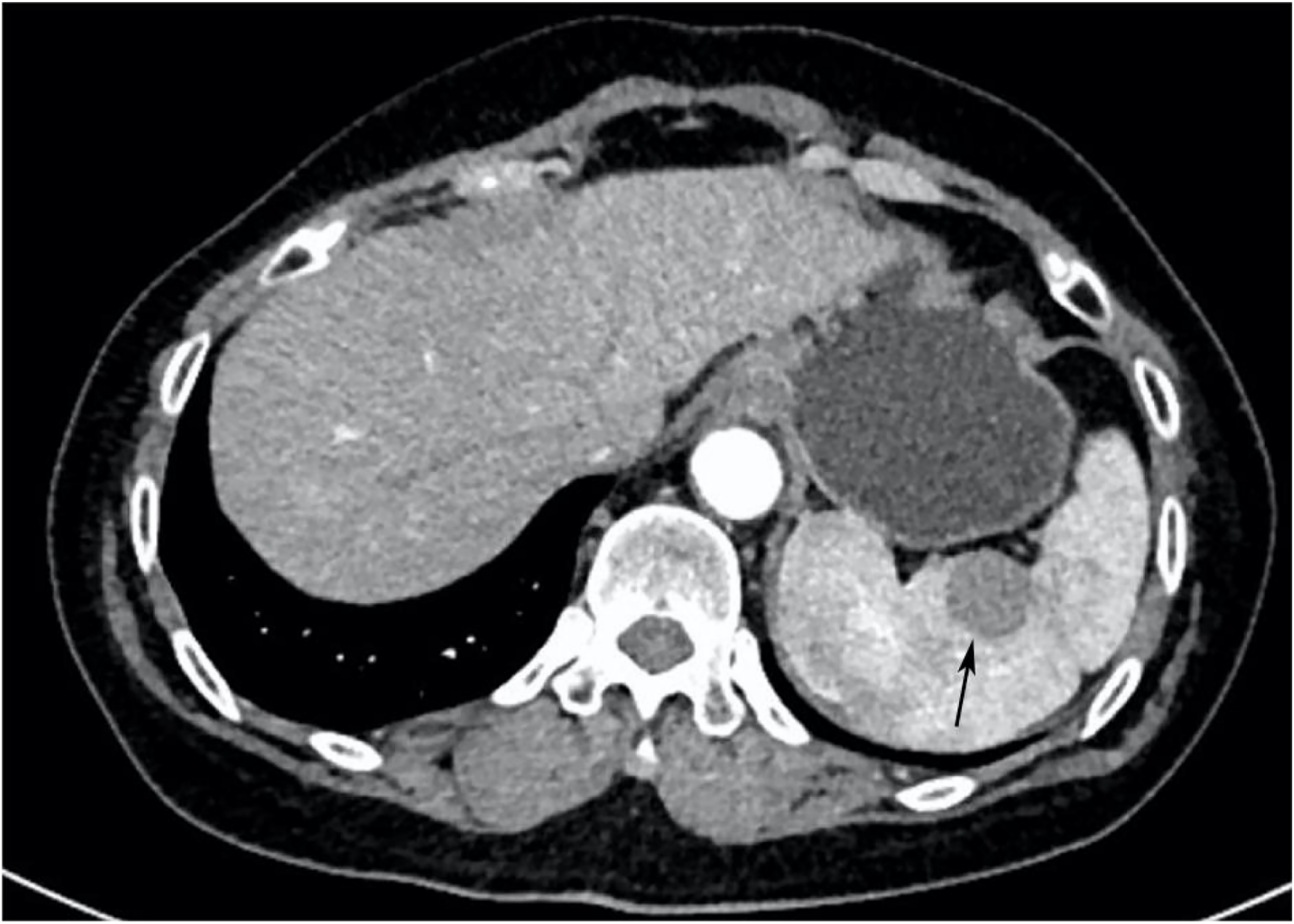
CT image. The spleen was enlarged and unevenly distributed; two isodense enhanced foci were observed in the spleen, with the larger one having approximately 20 mm diameter (indicated by the arrow).
